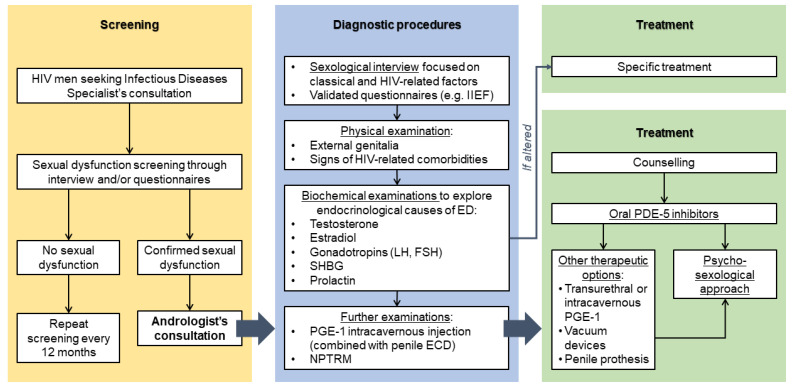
Diagnostic and subsequent therapeutic work-up to erectile dysfunction in HIV men. Abbreviations: IIEF, International Index of Erectile Function; ED, erectile dysfunction; LH, luteinizing hormone; FSH, follicle-stimulating hormone; SHBG, sex hormone-binding globulin; PGE-1, prostaglandin E1, ECD, eco-color-Doppler; NPTRM, nocturnal penile tumescence and rigidity monitoring; PDE-5, phosphodiesterase type 5.
